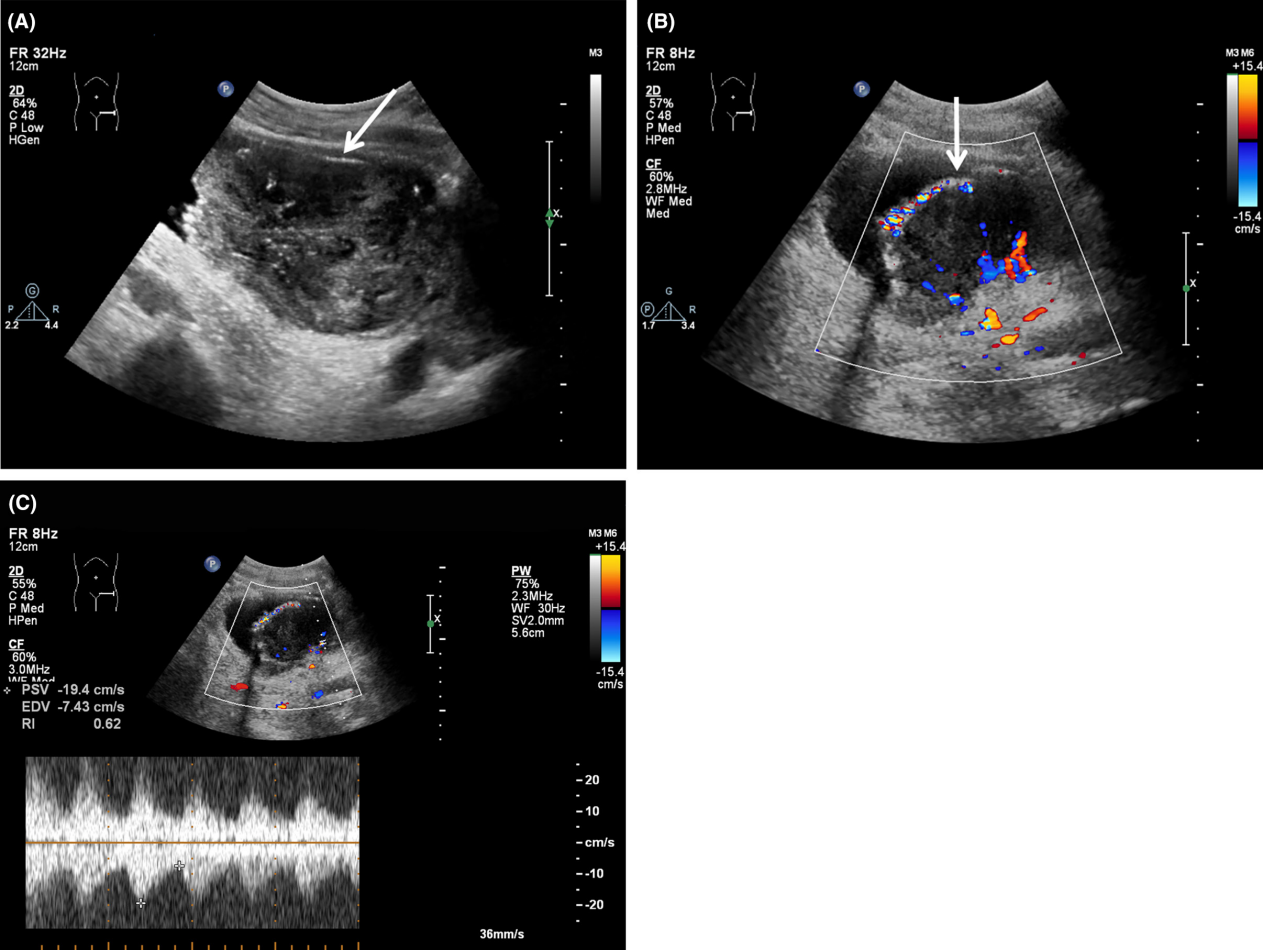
Abdominal ultrasound of sarcomatoid carcinoma of the urinary bladder. (A) Grayscale ultrasound showed a solid mass (arrow) with inhomogeneous internal echoes and hyperechoic periphery. (B) Color Doppler flow imaging showed abundant blood flow signals within the mass (arrow). (C) Pulse wave Doppler ultrasound showed a resistance index of 0.62.
